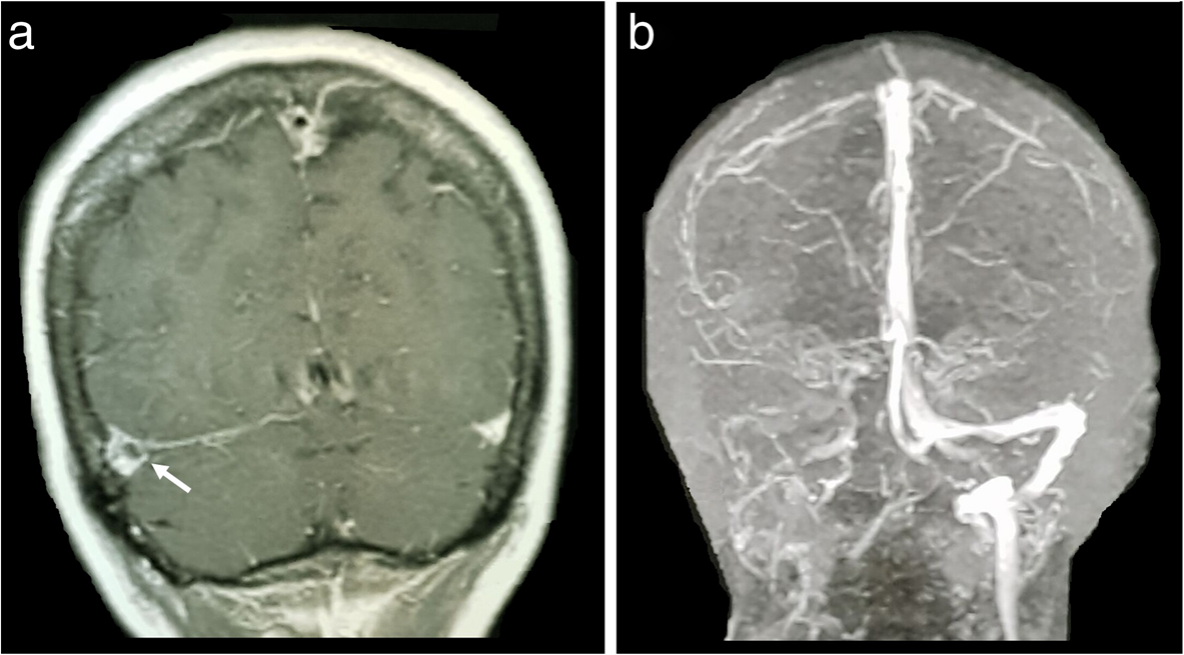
Coronal T2 MRI (a) demonstrating a filling defect in the right transverse sinus (white arrow). Maximum intensity projection (MIP) reconstruction of an MRI intracranial venogram (b) demonstrates complete non-opacification of the right transverse sinus. This was due to a chronic right transverse sinus thrombosis in a patient with myelofibrosis
